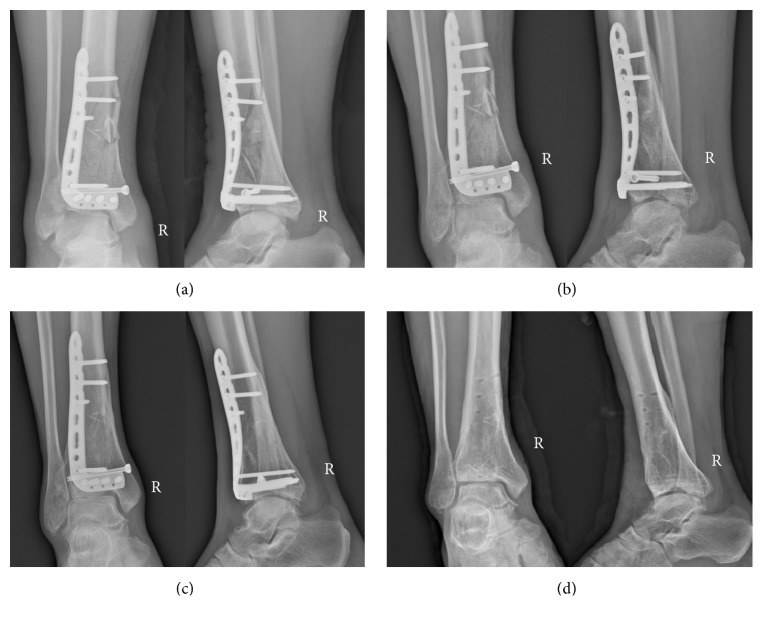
The radiography after the operation. (a) The anteroposterior and lateral X-ray after operation. (b) The anteroposterior and lateral X-ray at 3 months postoperatively. (c) The anteroposterior and lateral X-ray at 6 months postoperatively. (d) The anteroposterior and lateral X-ray at 18 months postoperatively (after internal fixation removal).
